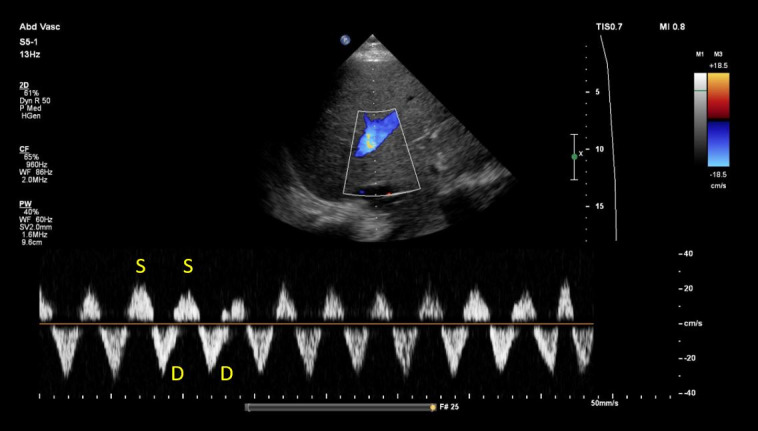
Hepatic vein Doppler demonstrating systolic flow reversal.
